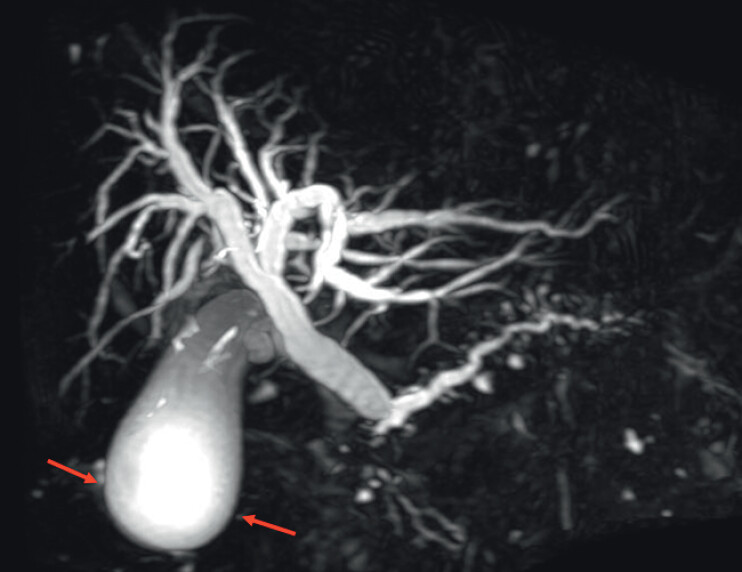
Magnetic resonance imaging revealed an enlarged gallbladder with wall thickening, indicating acute cholecystitis (red arrow).
